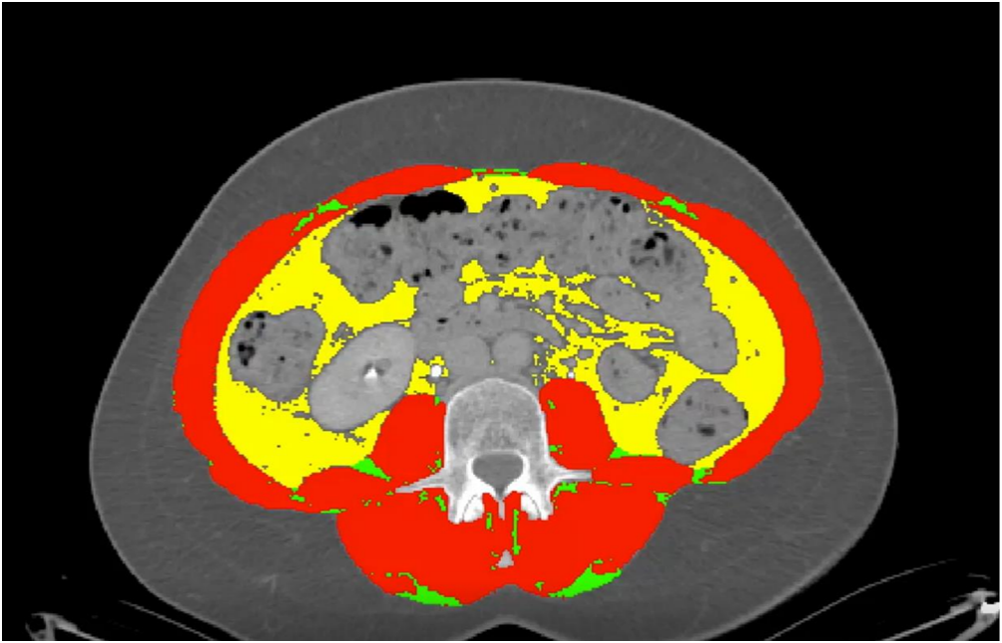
Axial CT slice at the mid‐L3 vertebral level segmented using Slice‐O‐Matic software and Horos, taken from Steele et al. guidance.^9^
